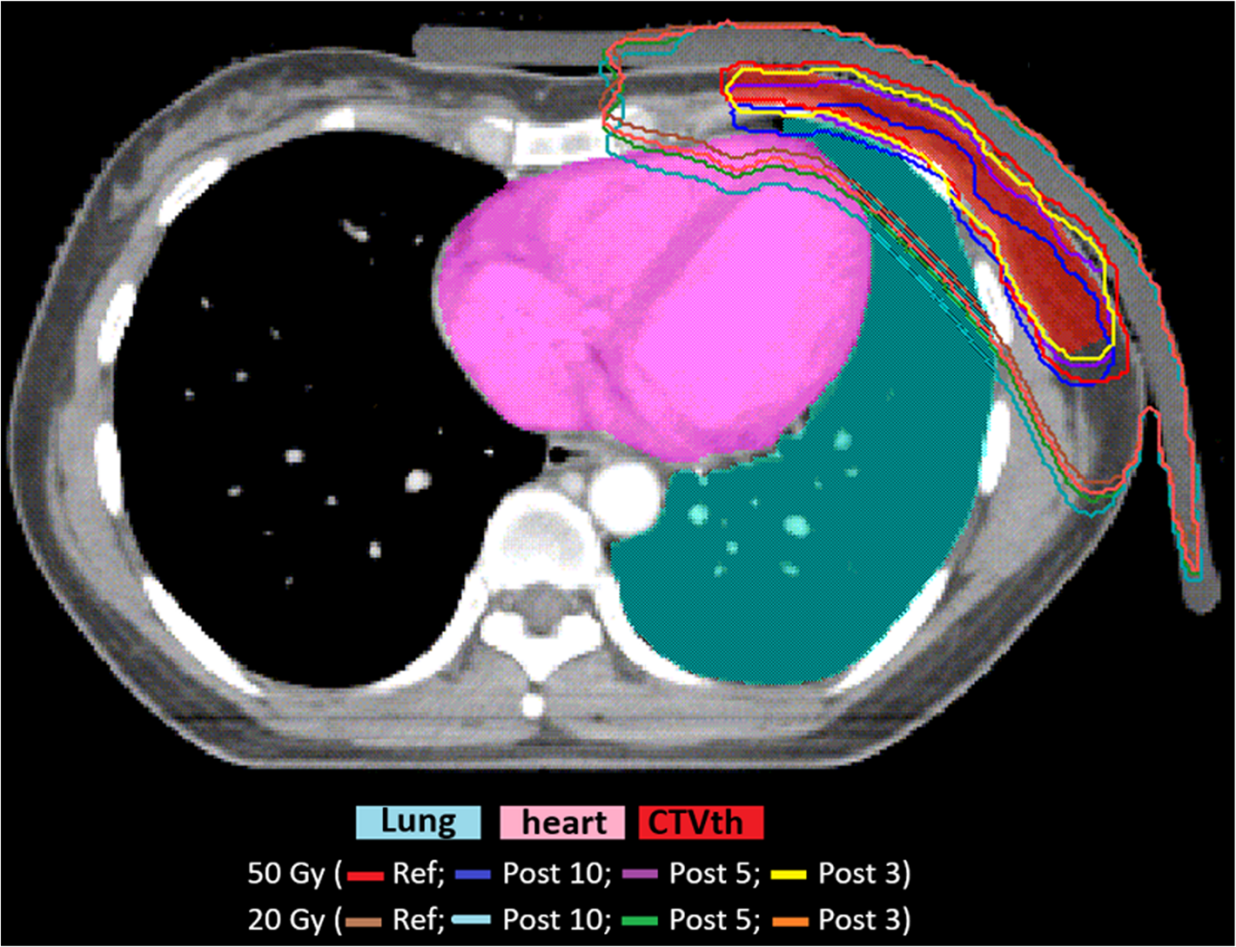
CT transversal scan of a representative patient with isodoses lines of the reference and corresponding perturbed plan. CT transversal slice of a representative patient with 50 Gy, and 20 Gy isodose lines of the reference plan (Ref) and of the corresponding plan perturbed with isocenter shifts in the posterior direction of 10, 5, 3 mm (Post 10, Post 5, Post 3)
